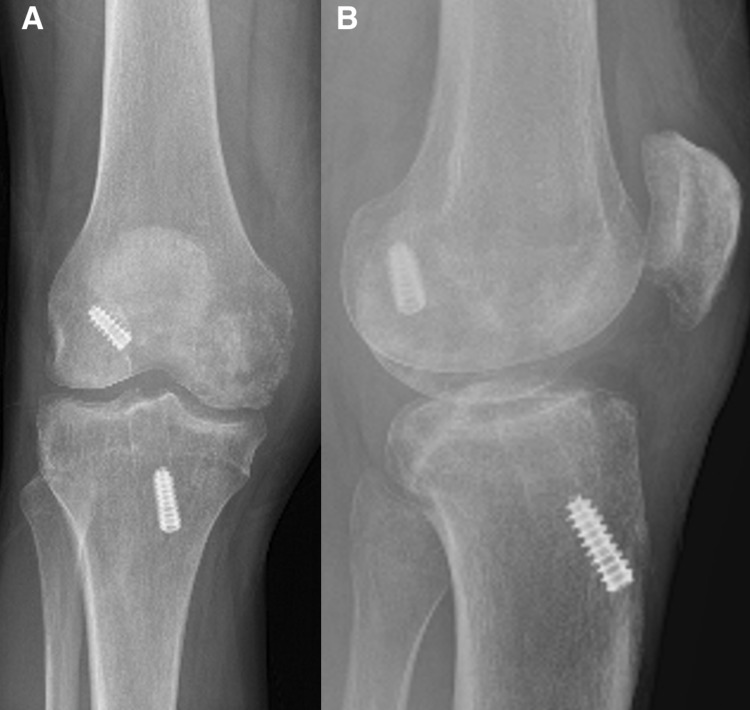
(a) Anteroposterior and (b) lateral radiographs of the right knee of a 29-year-old man (patient three) with previous anterior cruciate ligament reconstruction and a medial femoral condyle lesion.
